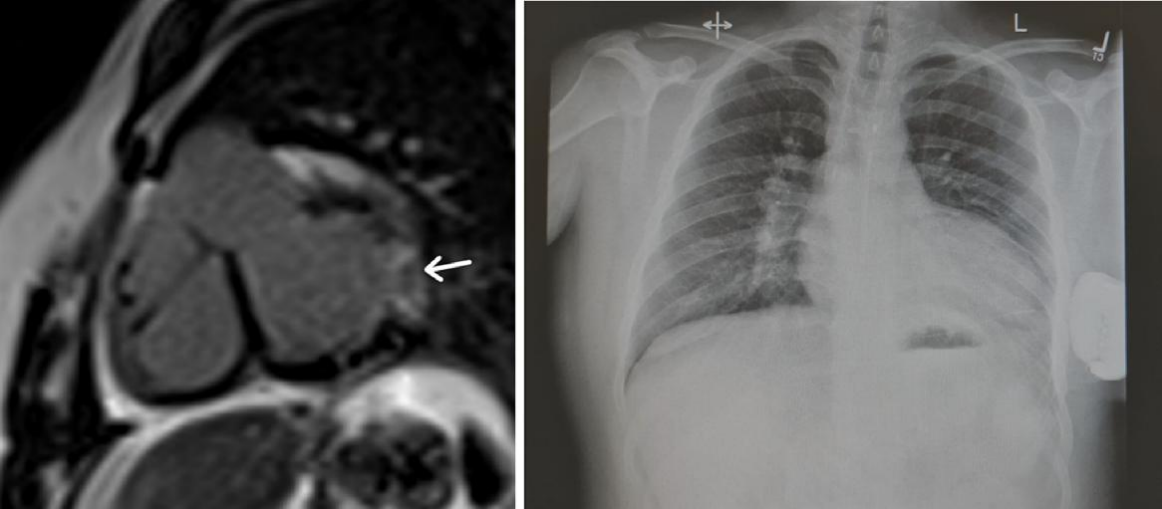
cardiac magnetic resonance [EF (50%)] showing late gadolinium enhancement in the basal anterolateral and inferolateral walls (white arrow) (left). Chest radiograph showing subcutaneous implantable cardioverter defibrillator insertion (right). *Source*: Mater Private Network.
